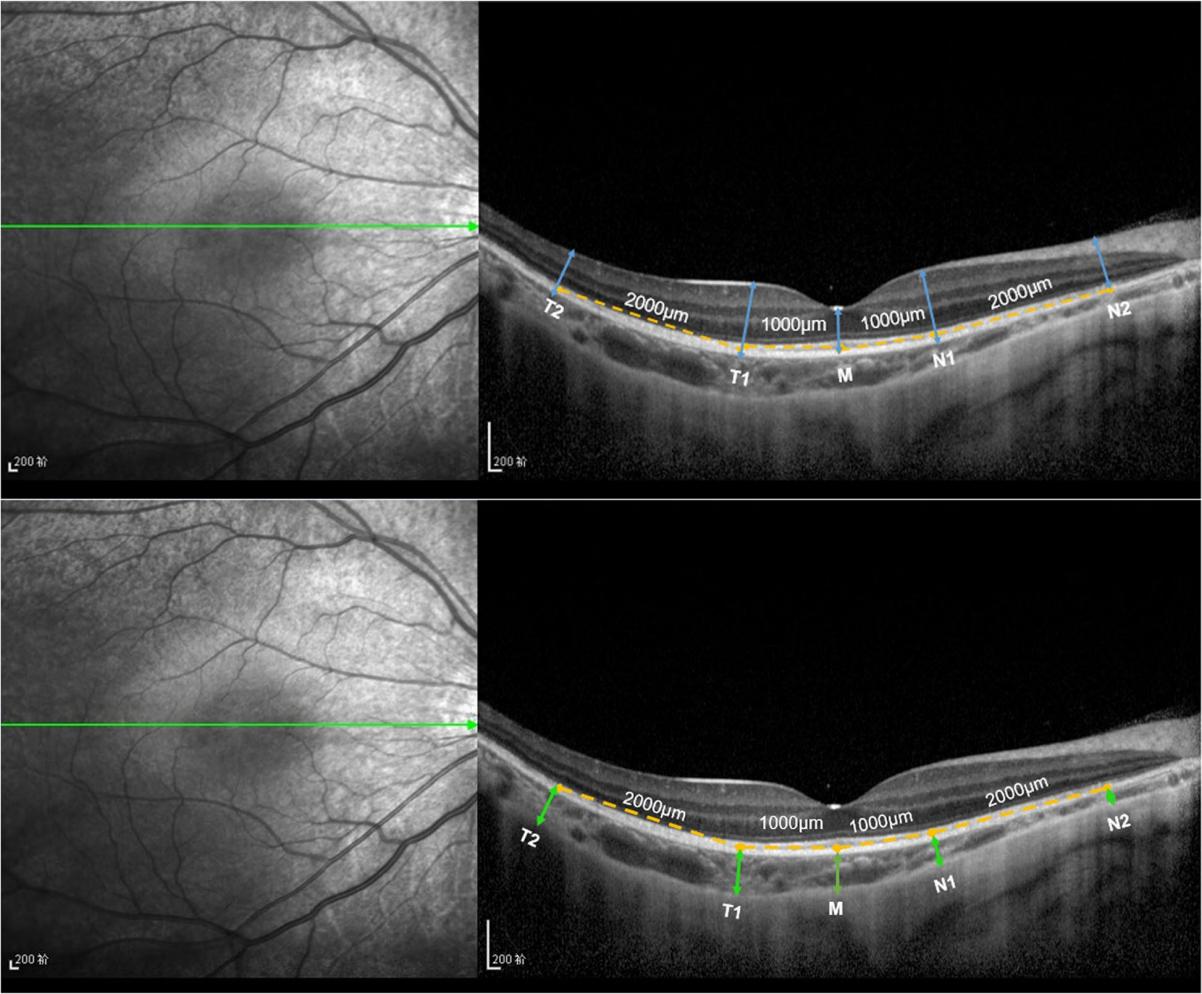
Measurement map of the thicknesses of the retina and choroid. The retinal thickness (RT) is the distance between the inner limiting membrane and the outer border of the RPE; the choroid thickness (CT) is measured from the outer border of the RPE vertically to the inner scleral border. M: foveal thickness; N1: thickness 1000 μm nasal to the fovea; N2: thickness 3000 μm nasal to the fovea; T1: thickness 1000 μm temporal to the fovea; T2: thickness 3000 μm temporal to the fovea
